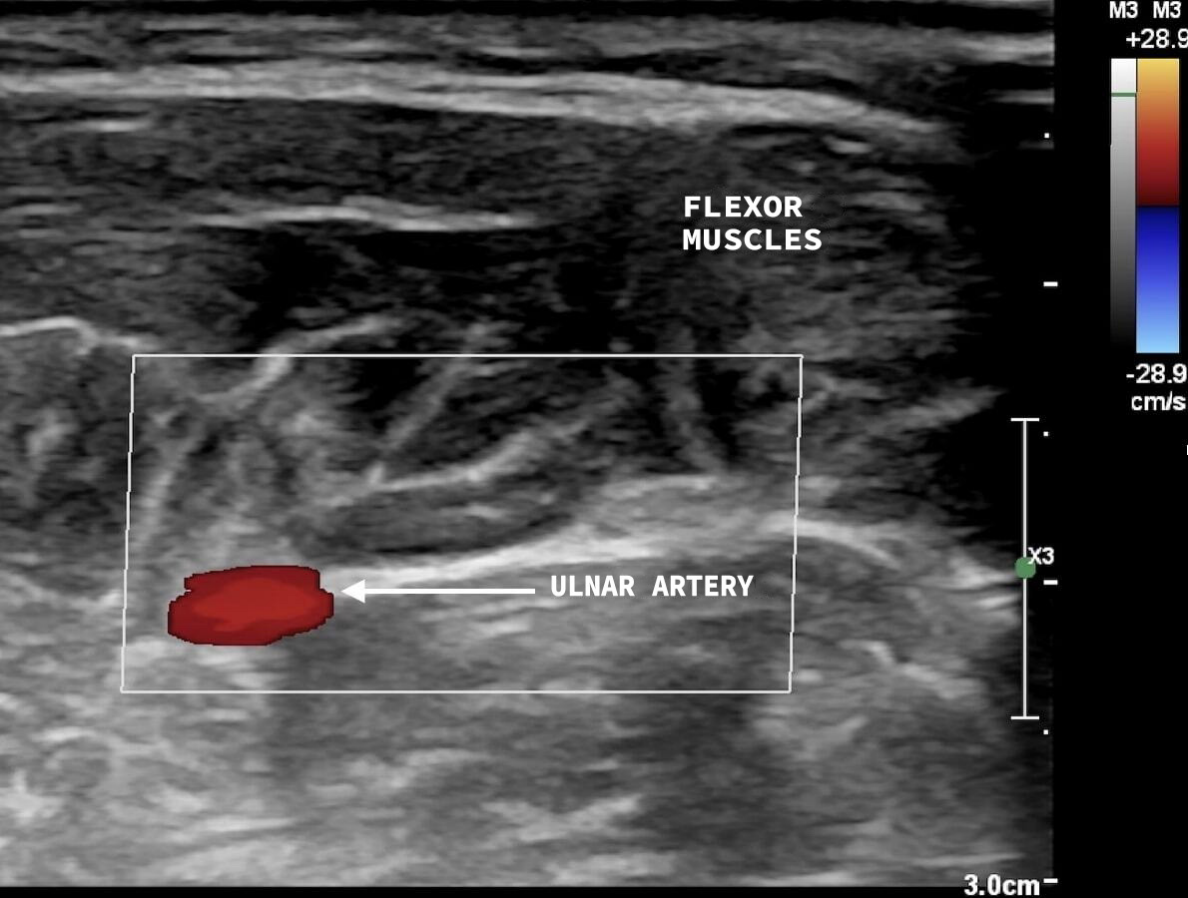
Vascular ultrasound, transverse view, with the ulnar artery following its normal path, coursing below the flexor muscles of the forearm.
